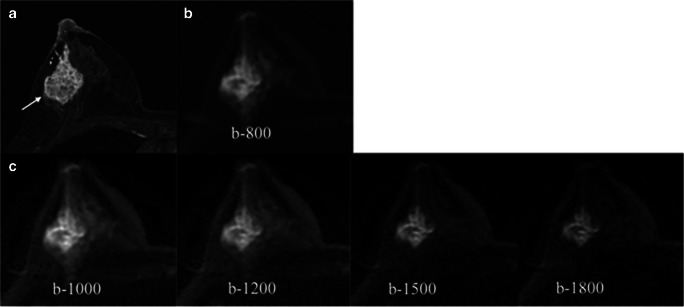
Axial images of a 47-year-old woman with dense breasts. Contrast-enhanced image (a) shows a 52-mm NMLE in the outer quadrants of the right breast. Biopsy proved a high-grade invasive ductal carcinoma. DWI acquired image (b) and synthetic images (c) are depicted. An increased lesion-to-normal-tissue contrast at synthetic high b-values due to suppression of the glandular signal is noticed. ADC mean value for this lesion was 1266 × 10^−6^ mm^2^/s. This lesion was correctly categorized as suspicious in both DWI and DCE-MRI. NMLE, non-mass lesion; DWI, diffusion-weighted imaging; ADC, apparent diffusion coefficient; DCE-MRI, dynamic contrast-enhanced magnetic resonance imaging
